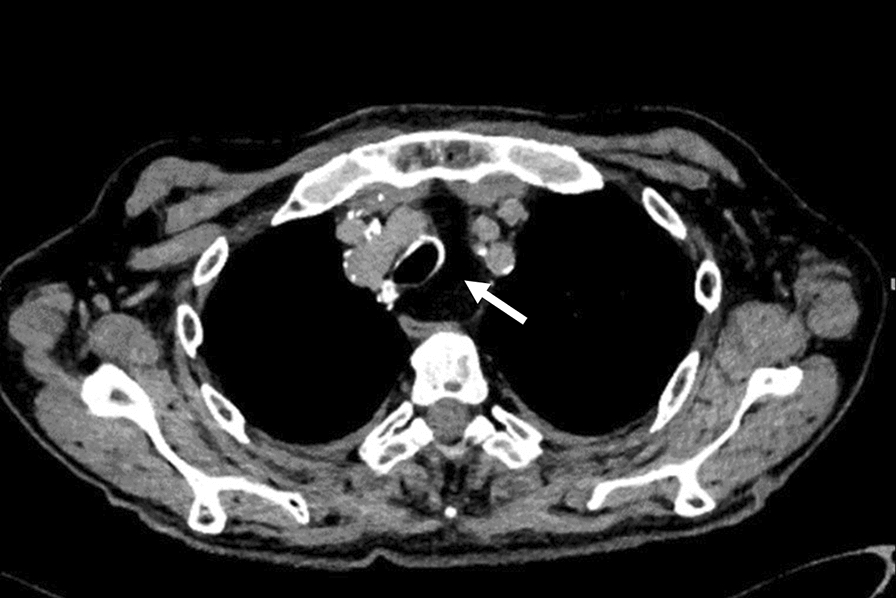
CT lymphangiography—transversal view, at the level of the mediastinal lipoma, as indicated by the white arrow
